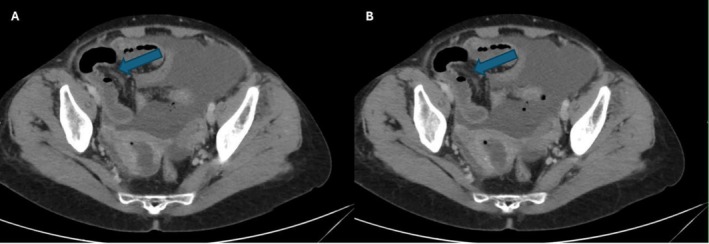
(A–B) Axial contrast‐enhanced CT images of the pelvis (10 March 2024) showing loculated intraperitoneal fluid with internal heterogeneous debris and small gas foci, with loss of posterior uterine fundal contour and a focal defect communicating with the collection.
